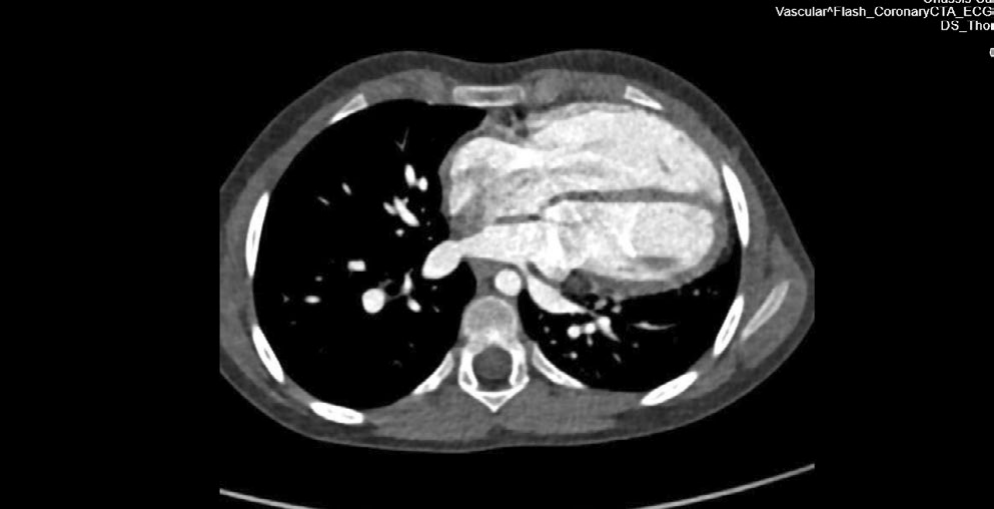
Preoperative computed tomography scan showing extreme levoposition.
